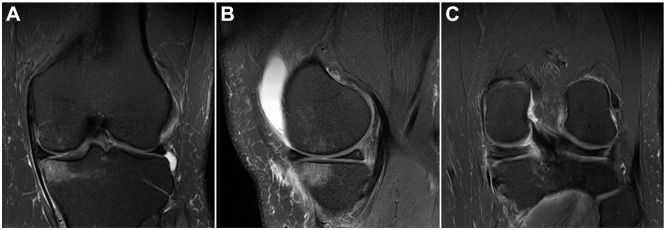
T2-weighted magnetic resonance imaging scans. (A) Coronal and (B) sagittal images showing a medial tibial bone marrow edema–like lesion. (C) Coronal image demonstrating a posterior medial meniscus root tear.
